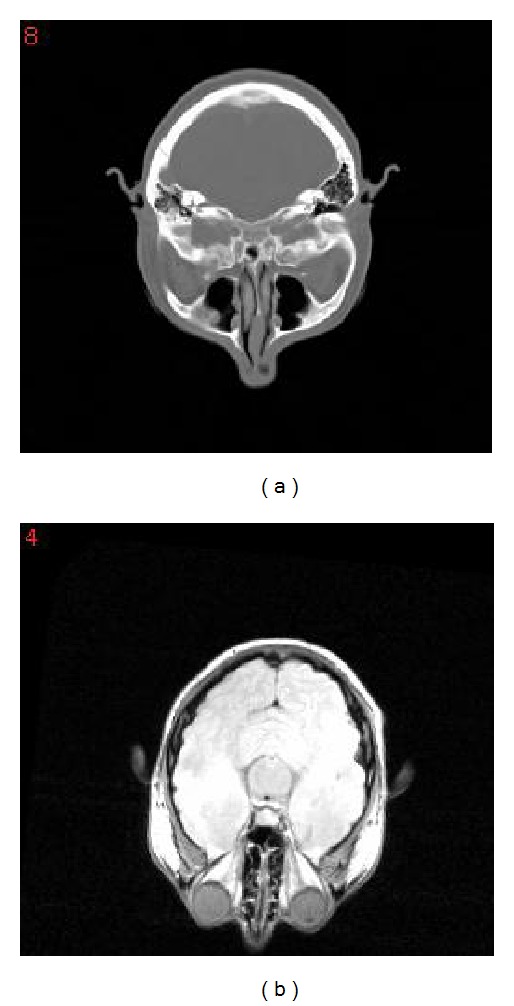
(a) A slice of CT image of Vanderbilt database; (b) a slice of MR image of Vanderbilt database.
